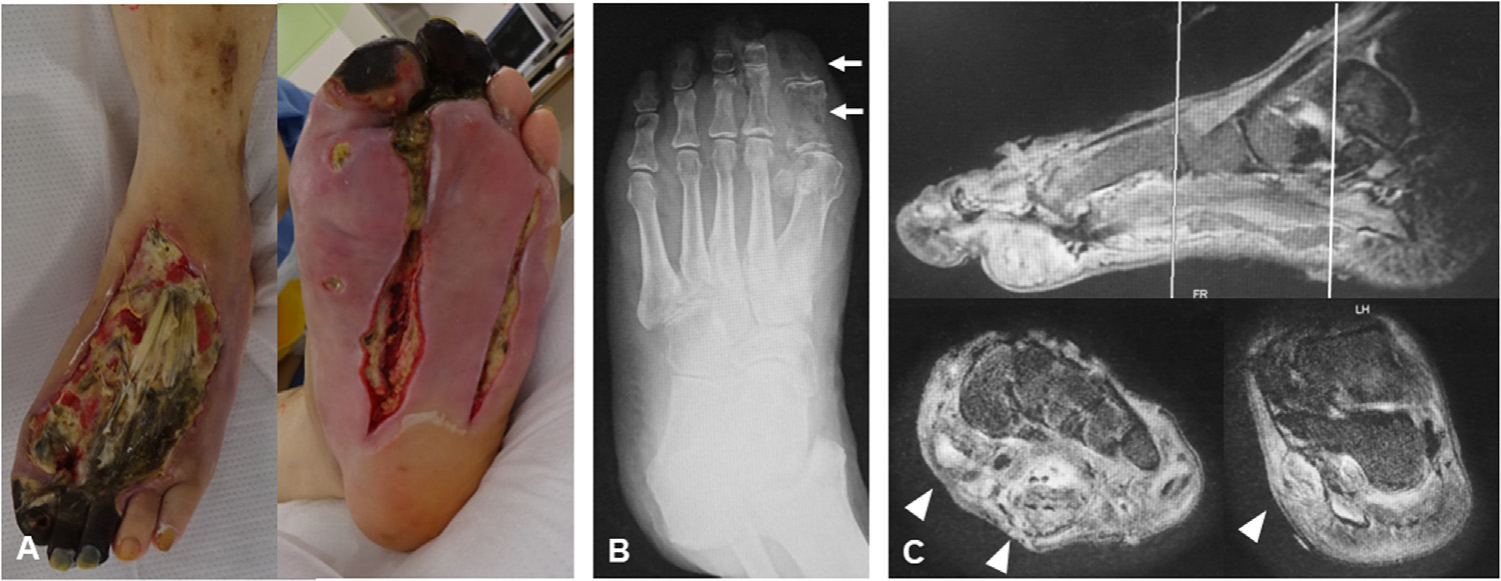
Infection control phase (case of a 78-year-old female with diabetic foot gangrene). (A) Photograph showing the initial external appearance at the first visit. Despite multiple debridements at another hospital, infection was not controlled, leading to a referral to our department for consideration of major amputation. (B) Radiograph highlighting osteolysis of the great toe bones (arrow). (C) MRI T2 fat-suppressed image demonstrating extensive infection along the plantar aspect of the foot, extending centrally along the flexor tendons (arrowhead).
